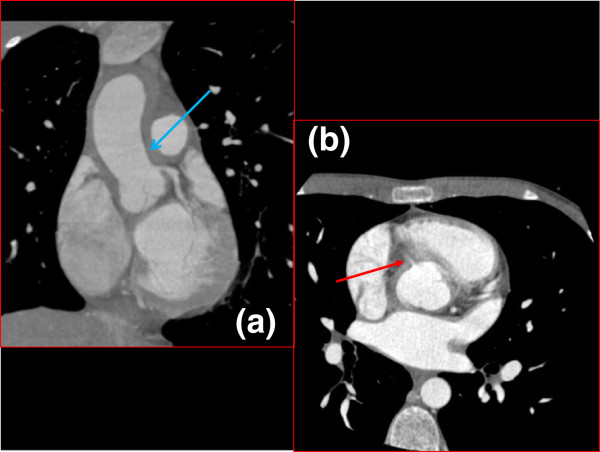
Coronal (a) and Axial (b) Cardiac Gated CT Chest. Fifty percent narrowing of the origin of the left coronary artery is demonstrated by arrow in (a, blue arrow). The right coronary artery should also be visible in this coronal section but was not due to stenosis (a). It can be seen as a wisp coming off the ascending aorta in the axial image (b, red arrow).
